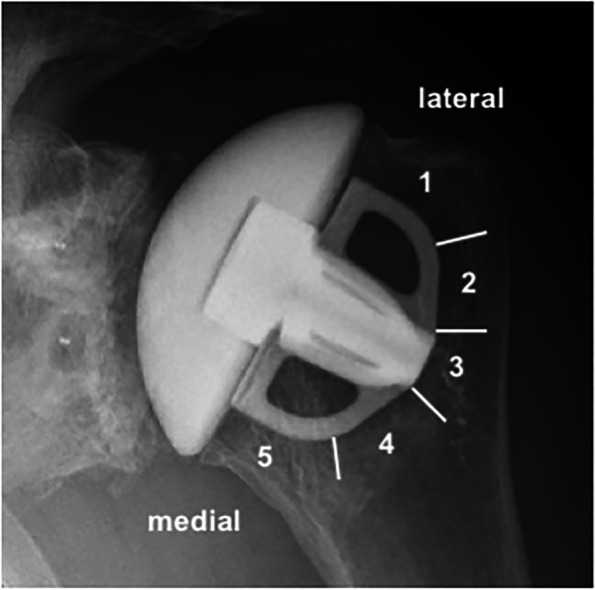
A radiograph demonstrates the humeral component of the prosthesis. The five zones were assessed for radiolucent lines around the humeral component or osteolysis at around the humeral head component
